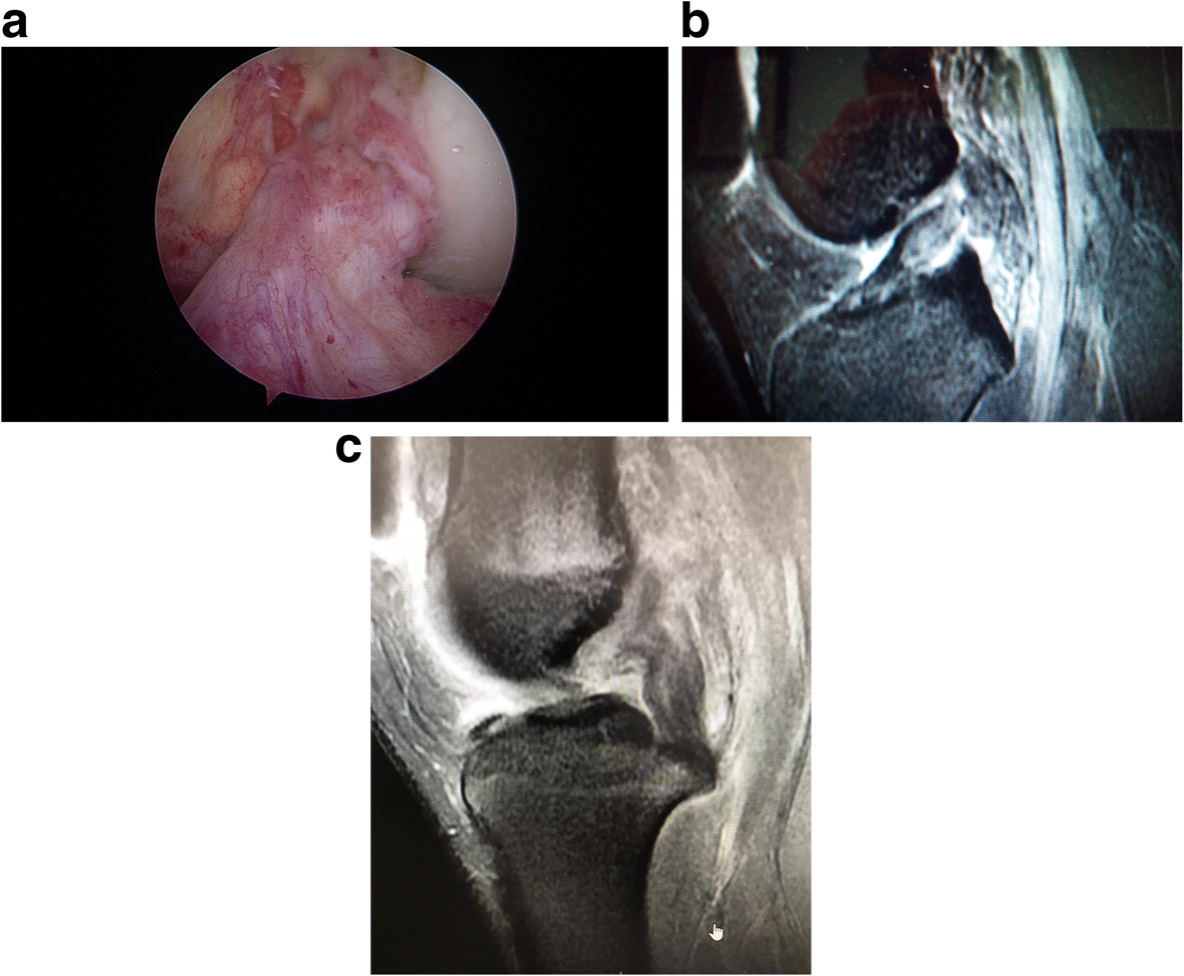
a ACL proximal rupture. b MRI proximal ACL rupture. c Proximal PCL rupture
